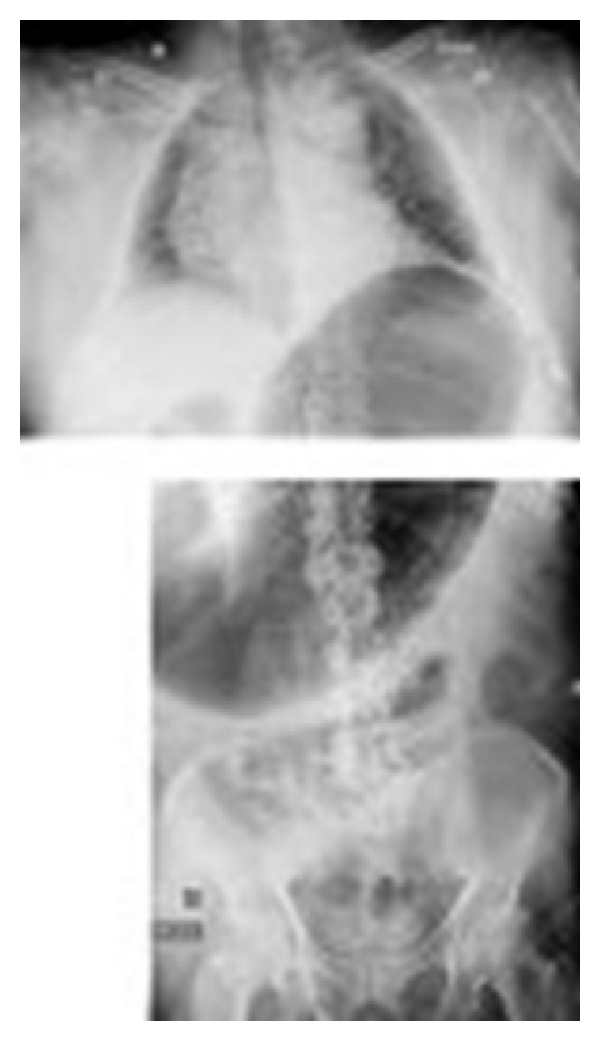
The first abdominal X-ray showing considerable gastric dilation.
